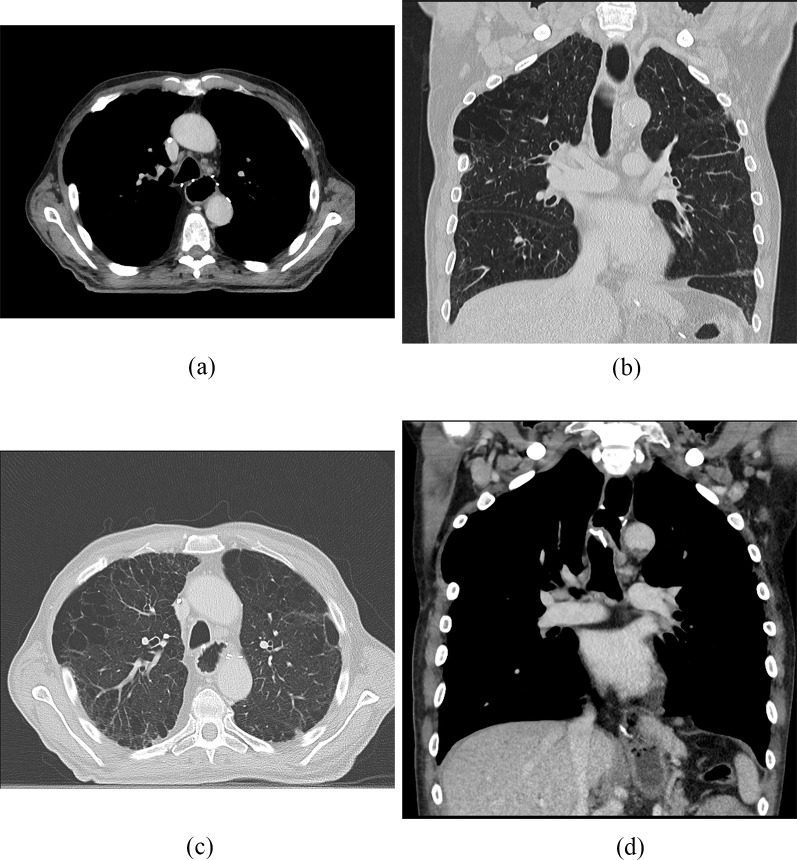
A-D Axial and coronal CT scans in soft tissue as well as in lung window showing a significant distance between third and fourth rib of the right hemithorax
